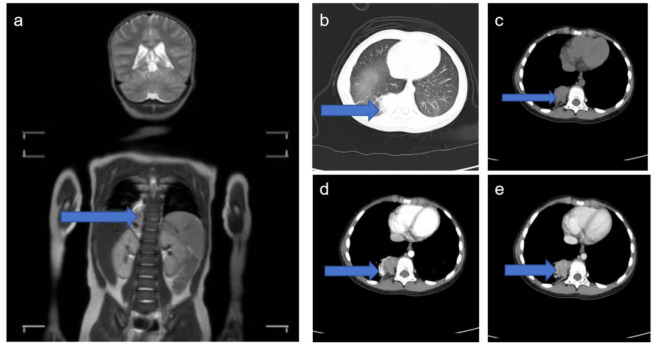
Imaging characteristics of the NF1 patient with GNB. (a) Whole - body screening MRI showing the space - occupying lesion in the right mediastinum (arrows). (b, c) Non - contrast chest CT images demonstrating the right mediastinal mass (arrows). (d, e) Contrast - enhanced chest CT images showing the right mediastinal lesion with heterogeneous enhancement (arrows).
